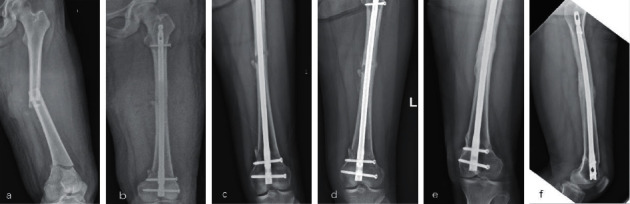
Female, 33, multi-fragment fracture of left femur with AO classification A3 in shaft, and A1 in distal part (a). Treated with retrograde nailing and postoperative FIB, 1-day postoperative radiograph (b). 1 month (c), 3-month (d) follow-up radiographs. 9-month follow-up radiographs indicated union (e, f).
